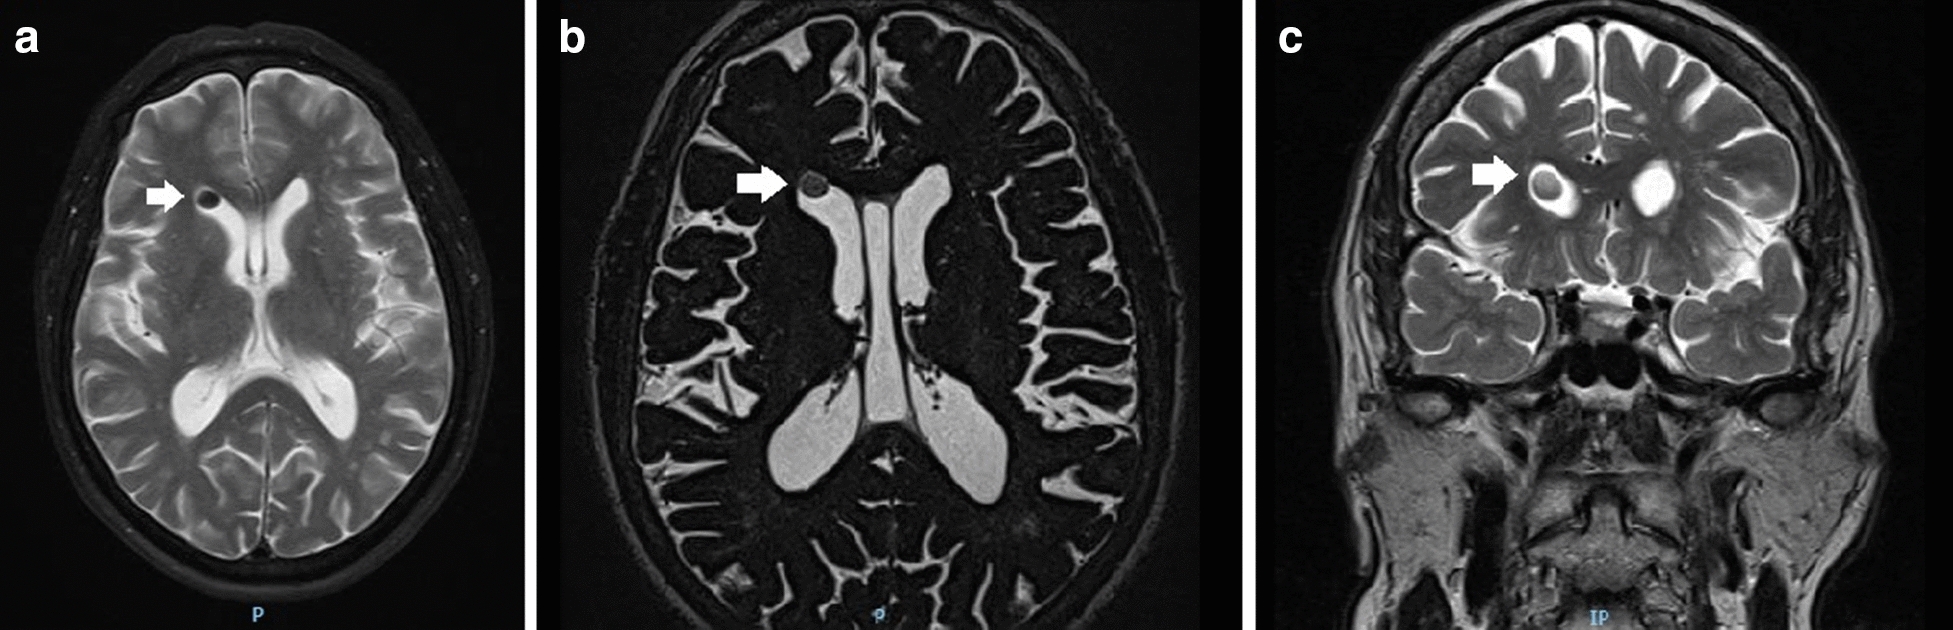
These are MRI T2 weighted
